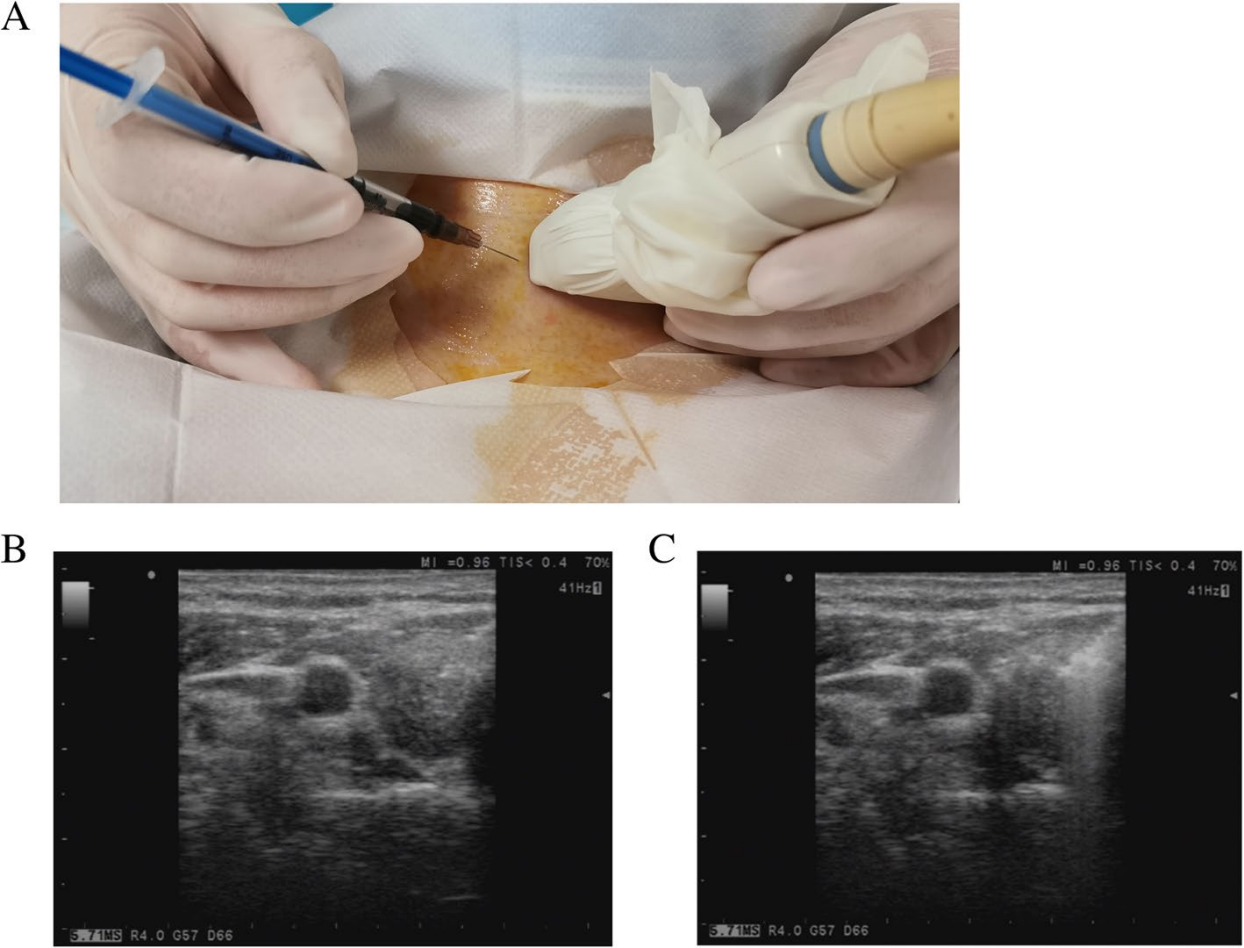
Preoperative ultrasound-guided CN suspension injection. A CN suspension was injected by using a 1-ml syringe. B Before the CN suspension injection, the needle can be seen under ultrasound guidance. C After the CN suspension injection, a high-echo area was formed in the thyroid gland
